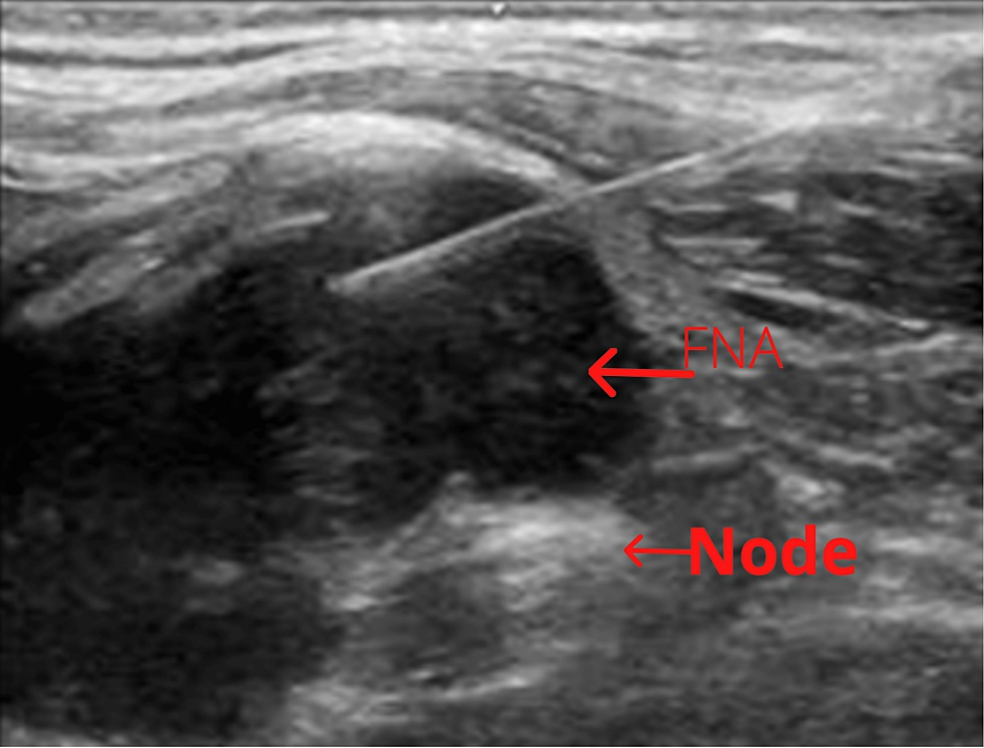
Ultrasound-guided fine-needle aspiration of the left supraclavicular lymph node.
